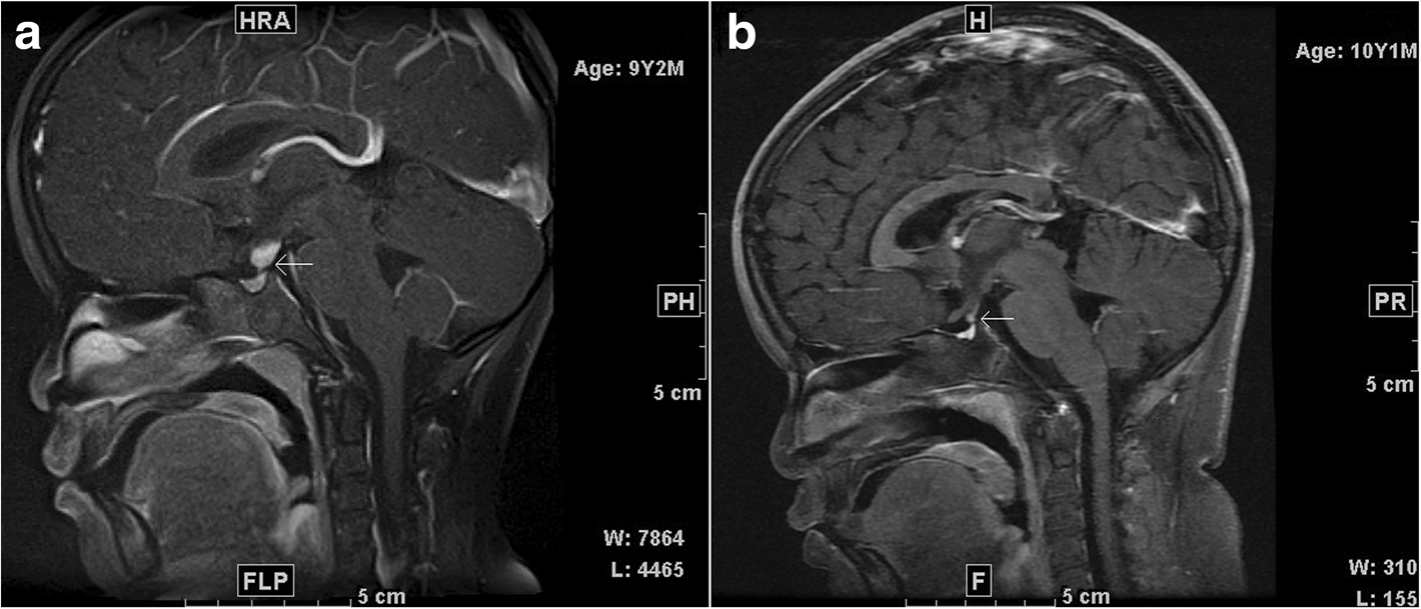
a. MRI scan of the lesion (*arrow*) in a 9-year-old child (case 1) before diagnosis demonstrated that the upper pituitary stalk was thickened, and a well-circumscribed and regular homogeneous enhanced mass was observed (sagittal T1 contrast-enhanced images). b. MRI scan of the lesion in case 1 at 11 months of follow-up demonstrated that the pituitary stalk (*arrow*) was fine and the homogeneous enhanced mass had regressed, but the anterior pituitary narrowed (sagittal T1 contrast-enhanced images)
